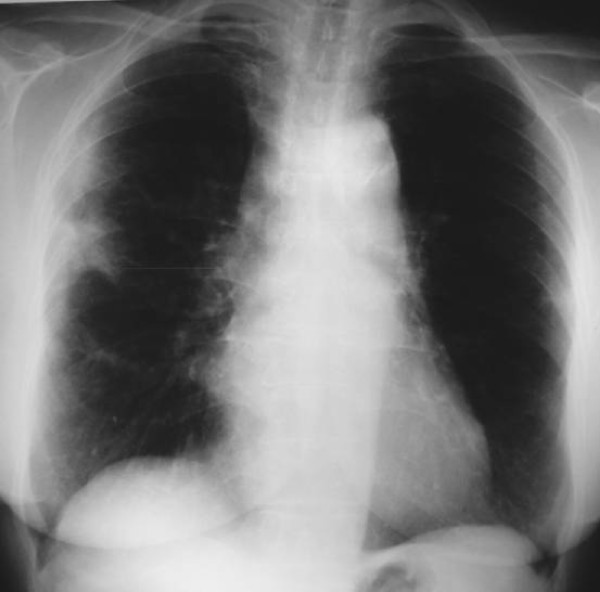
Posteroanterior chest radiograph at presentation shows patchy areas of consolidation with a predominantly peripheral and subpleural distribution mainly in the right lung.
